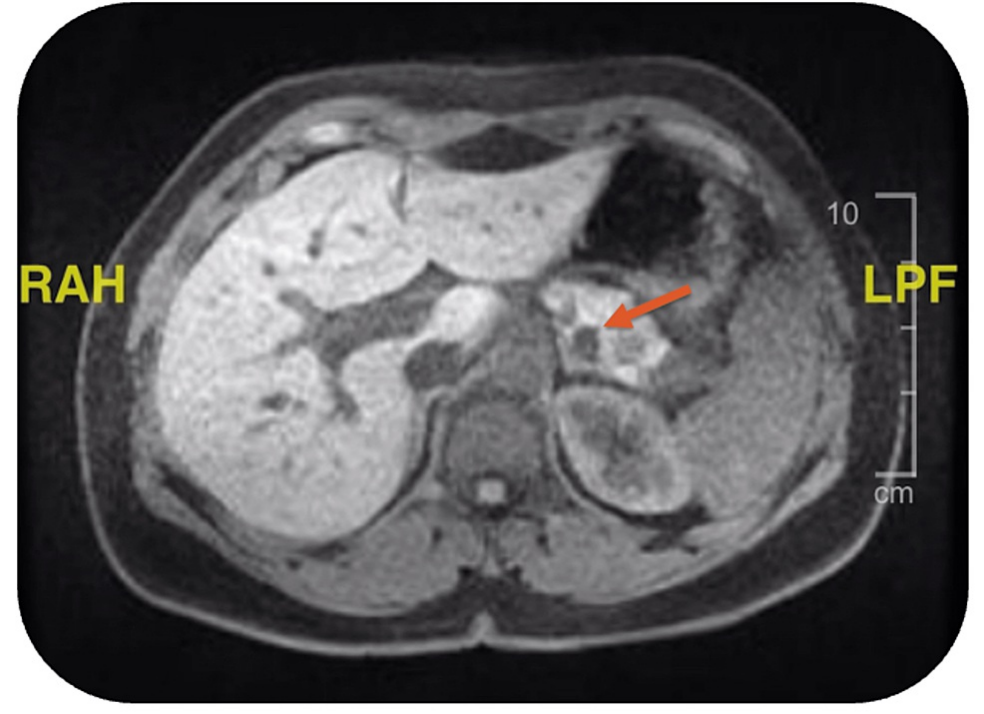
Abdominal MRI T1 pre-contrast imaging demonstrating 13 mm focal area of lower signal intensity in the tail of the pancreas (orange arrow). LPF = Low Pass Filter, RAH = Radiological Axis System
